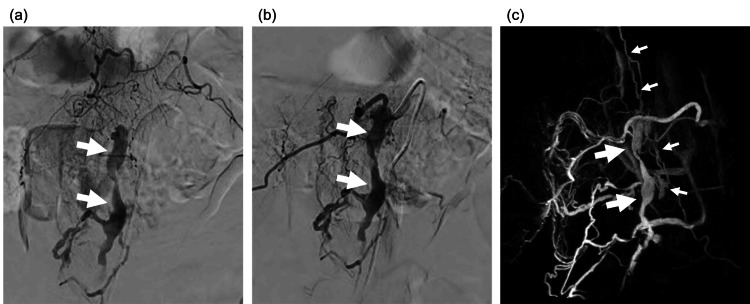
Spinal digital subtraction angiography, the right T12 (a) and L1 (b) injection, showed a spinal extradural arteriovenous fistula with the extradural venous pouch (arrows), which is the target for embolization. Volume rendered image of the CT angiography (c) confirms that the venous pouch (arrows) is connected to the radiculomedullary and perimedullary veins (small arrows), demonstrating the abnormal venous drainage pattern that causes congestive myelopathy.
